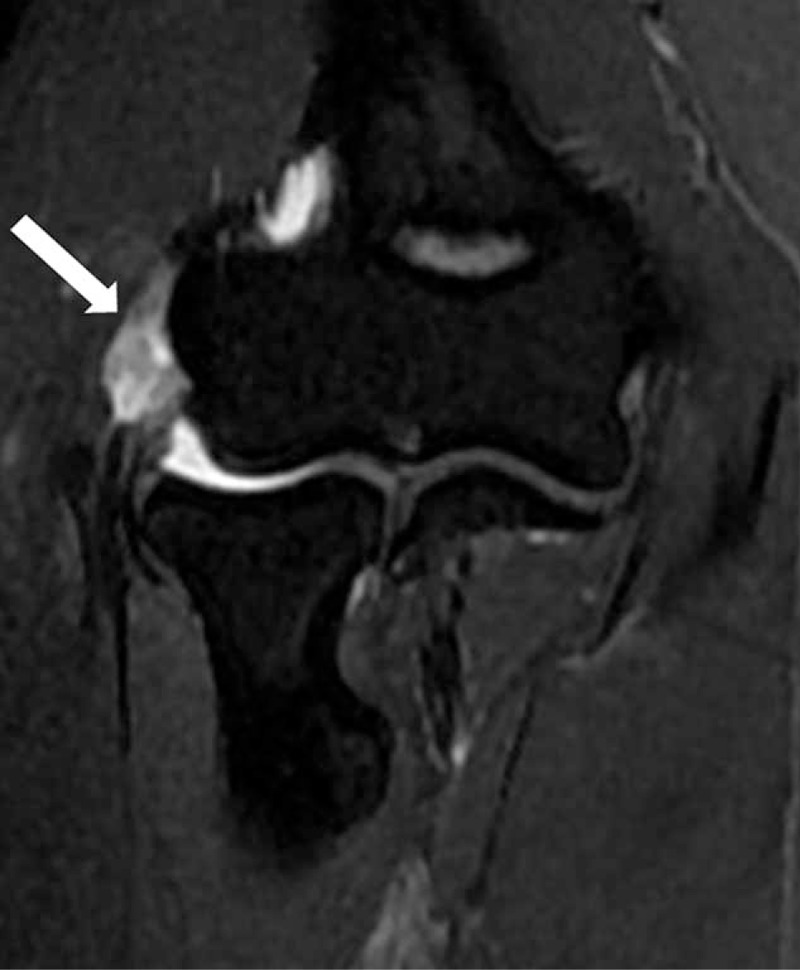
40-year-old woman with right elbow pain ∼2 years (Tendinopathy score = 3; PRTEE score = 89). Coronal T2-weighted fat-suppressed MR image shows a generalized increase in the tendon signal (white arrow). PRTEE = Patient-Rated Tennis Elbow Evaluation.
